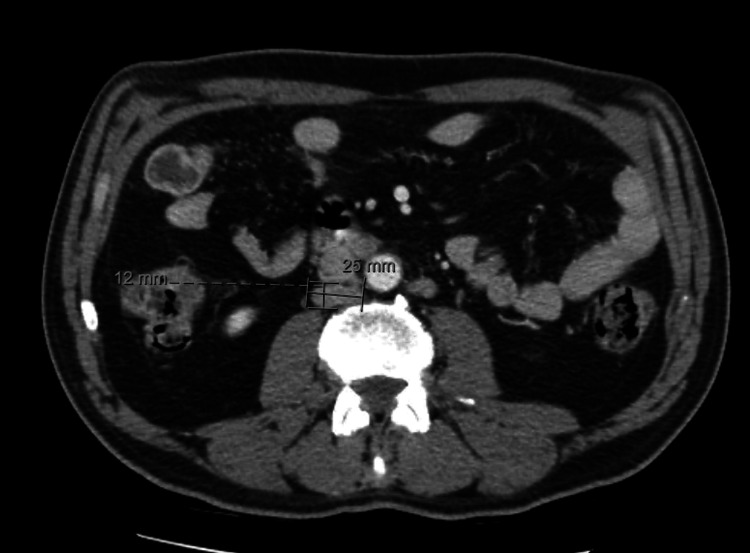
Staging CT scan showing multiple enlarged lumboaortic lymph nodes (Case 2) The largest measured 25 × 12 mm in the retrocaval region.
